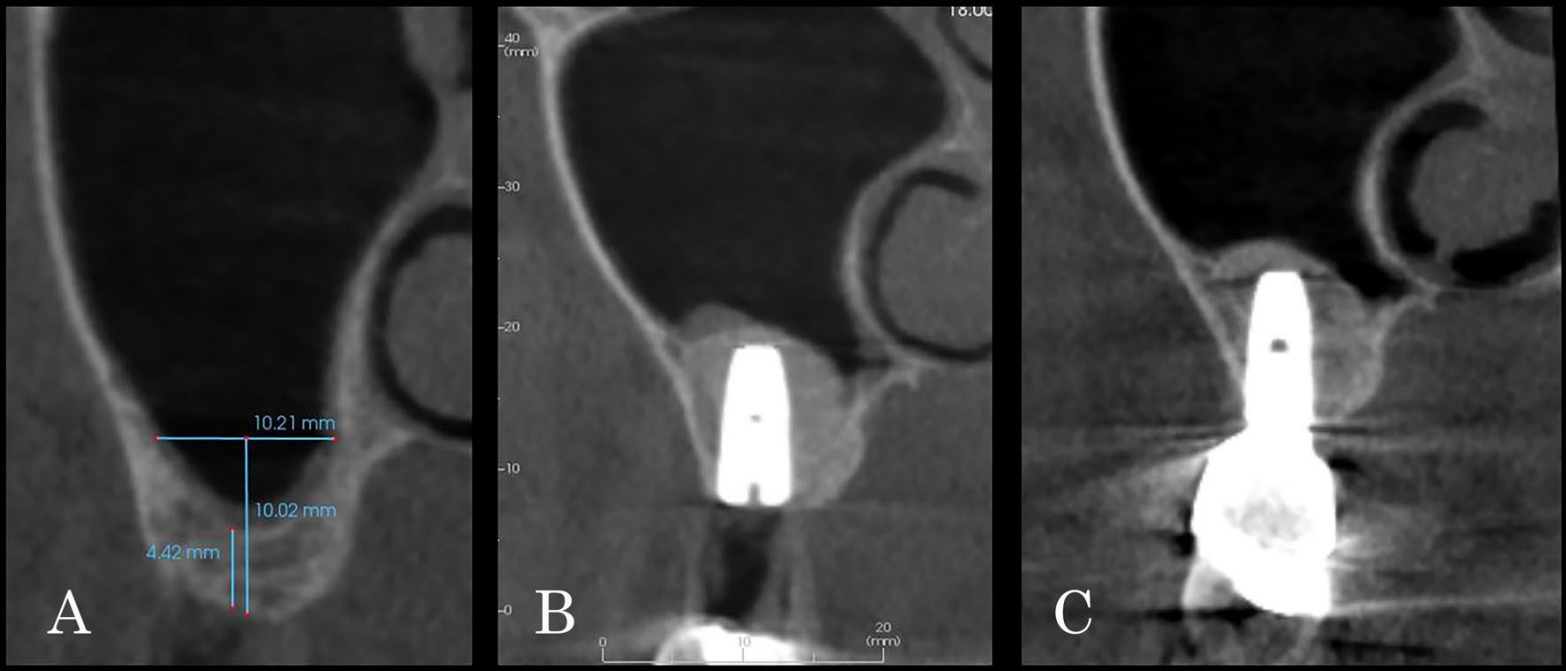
CBCT cross-section images taken presurgically (A), at T0 (B) and T1 (C) showing the “tent-pole” effect of the implant apex
